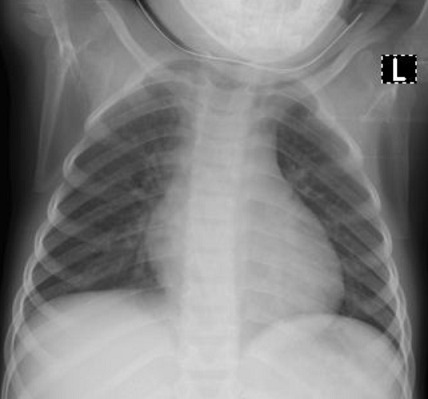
Patient two chest radiograph showing reticular opacities in the paracardiac and paratracheal regions with sparing of the lateral lung fields
